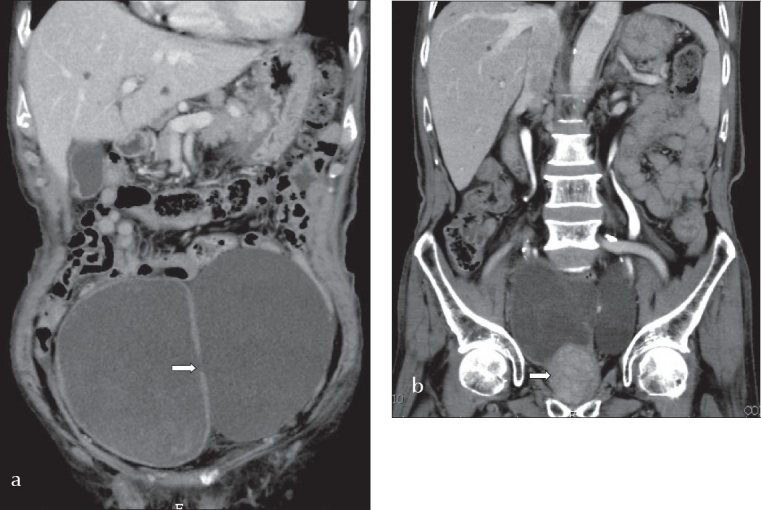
CT scan of the pelvis (a) showing a bladder diverticulum (left) connected with the urinary bladder (right) by a small hole (arrow). The large prostate is also demonstrated (arrow) (b).
